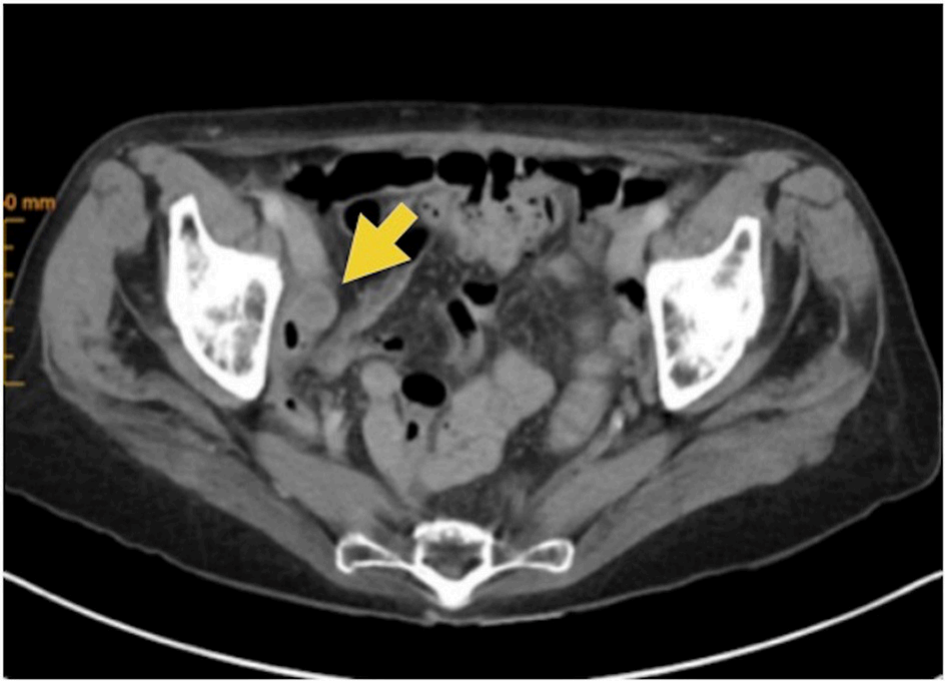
Metastatic suspected lesions in CT scan (marked with arrow).
